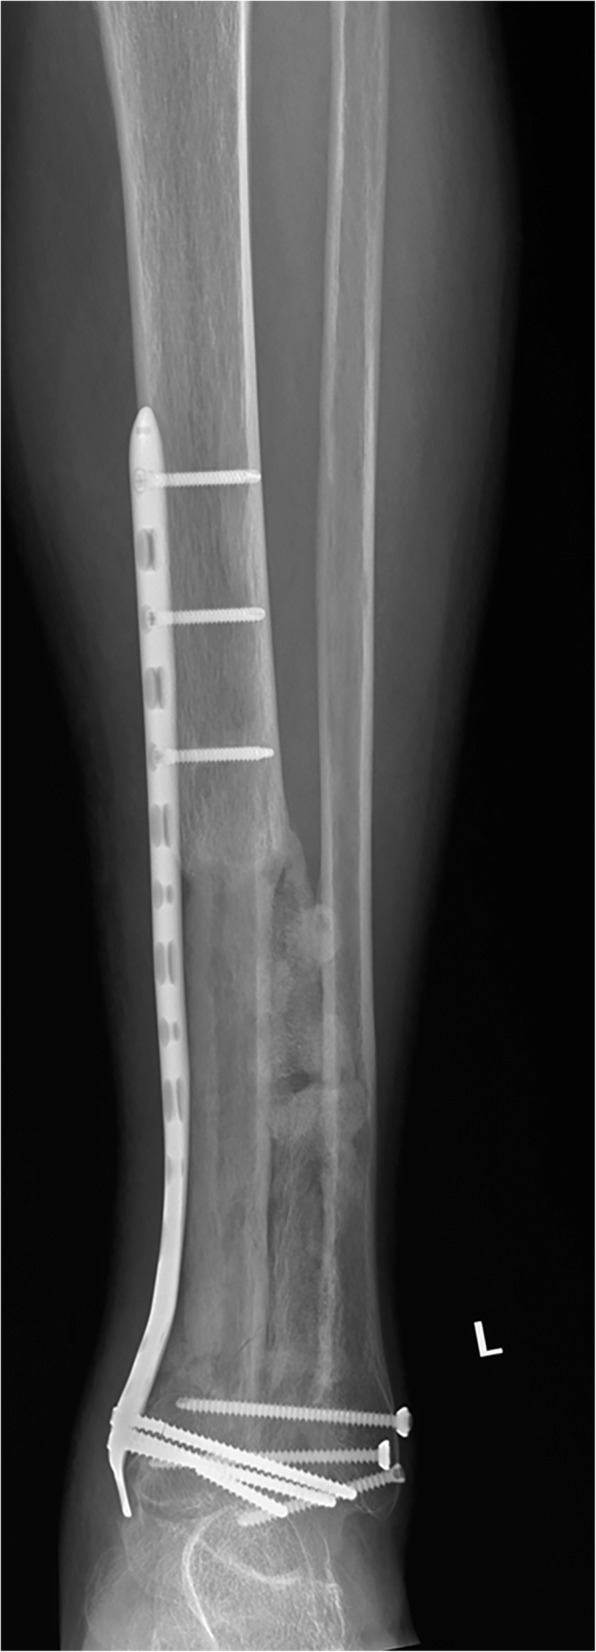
Plain radiograph taken 22 months after surgery showing that the fibula transfer is becoming hypertrophy under weight-bearing stimulation (Patient 6)
